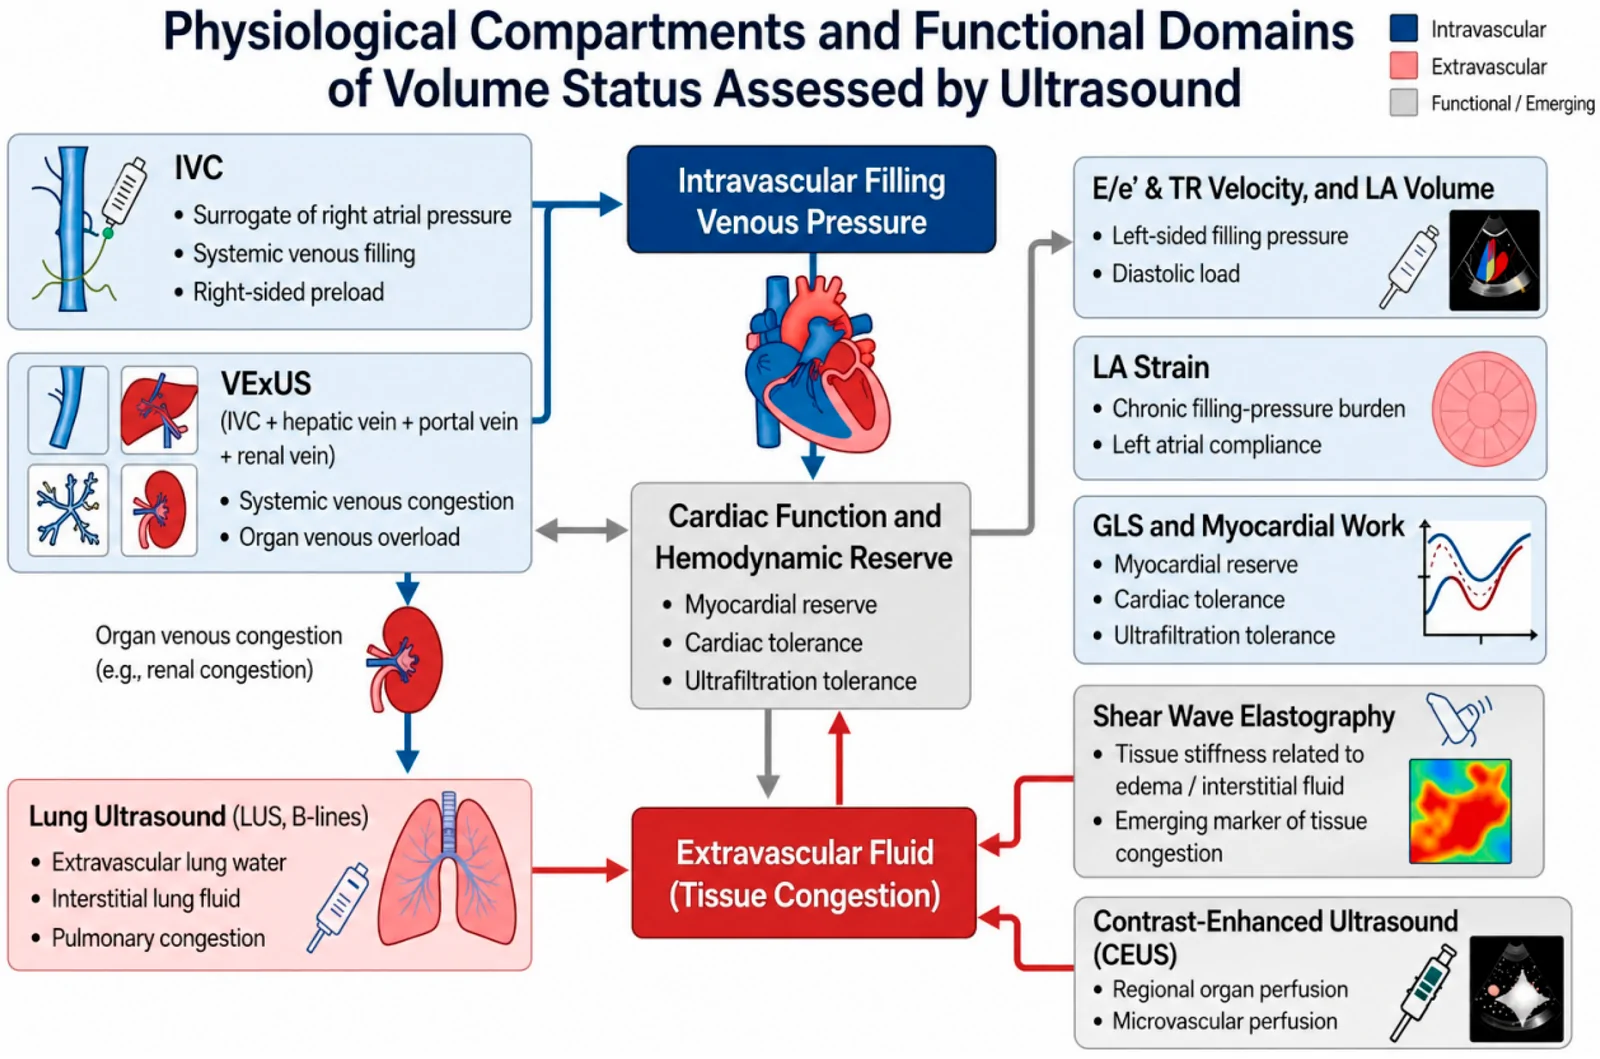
Compartment-specific framework for multimodal ultrasound-based volume assessment in maintenance hemodialysis. The figure summarizes how ultrasound-based techniques assess pulmonary congestion, intravascular filling, systemic venous congestion, cardiac reserve, tissue congestion, and organ perfusion. This framework emphasizes that dialysis volume assessment should move beyond single-parameter interpretation toward integrated evaluation of congestion, perfusion, cardiac reserve, blood pressure instability, and ultrafiltration tolerance.
